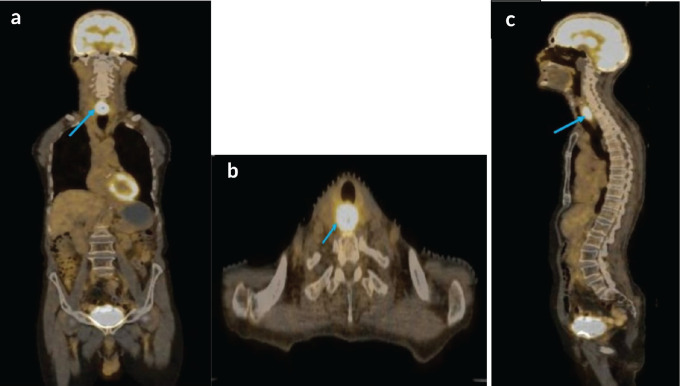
(a–c): Coronal axial and sagittal views respectively, of staging PET-CT scan showing FDG avid, cervical esophageal mass, with normal uptake seen over brain.
